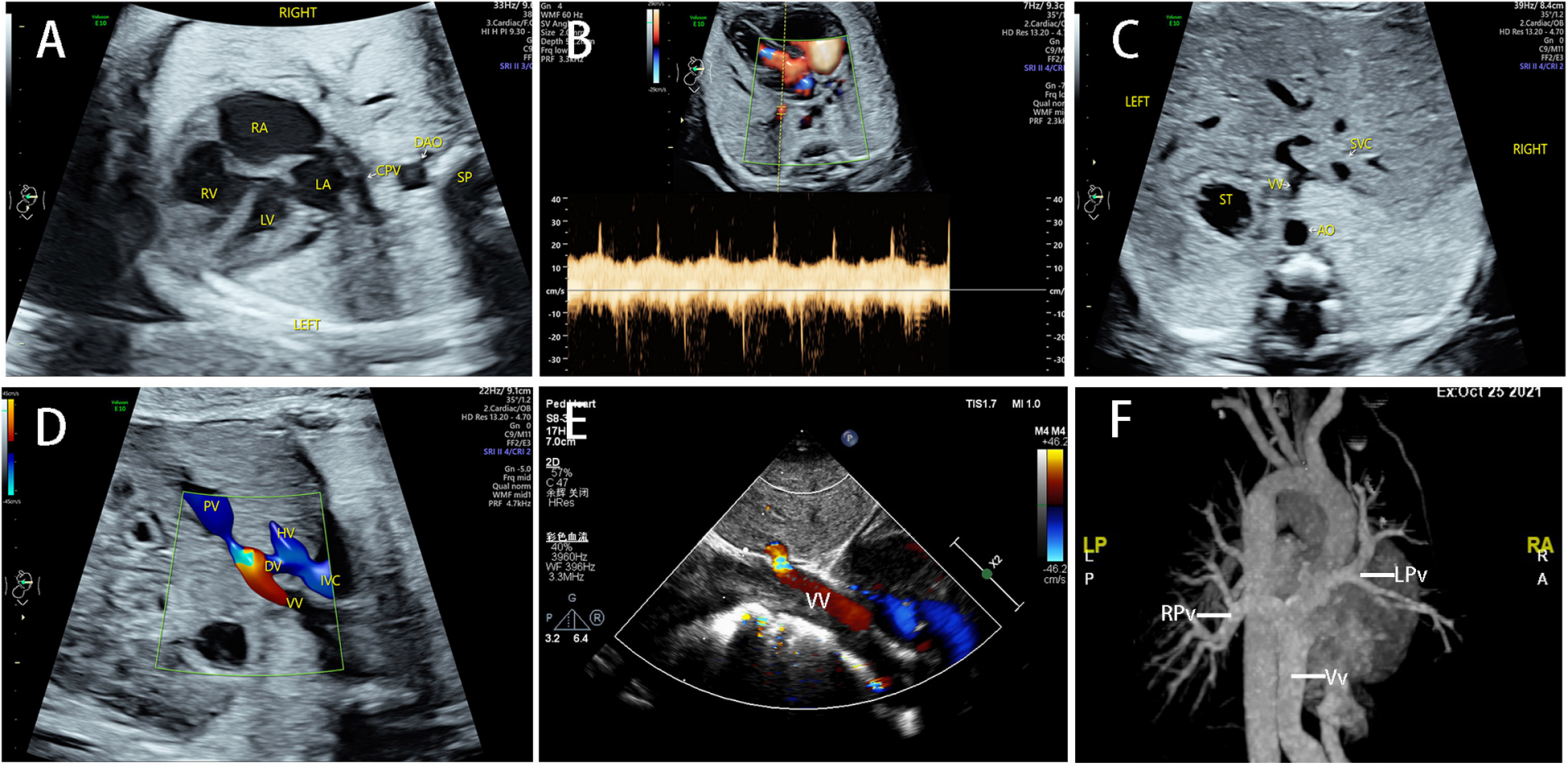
The sonographic features of infracardiac TAPVC and postnatal confirmation. (A) CPV was located behind the LA, with an increased posterior left atrium space. (B) The Doppler spectrum of PV was continuous instead of pulsatile. (C) The VV appeared as an extra vessel in the transabdominal section. (D) The VV drained into DV in a turbulent flow. (E) The obstruction was confirmed postnatally. (F) The CTA showed the entire drainage route of PVs. TAPVC, total anomalous pulmonary venous connection; PV, pulmonary vein; LA, left atrium; CPV, common pulmonary vein; VV, vertical vein; DV, ductus venous; CTA, computerized tomographic angiography.
